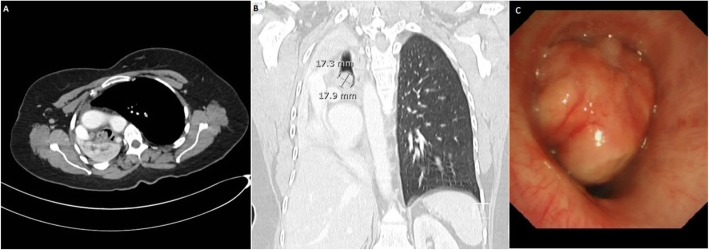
a and b Preoperative CT chest showing a right bronchial mass in the right main bronchus with total lung collapse and ipsilateral mediastinal shift. c: Bronchoscopy showing the mass occluding the right main bronchus
